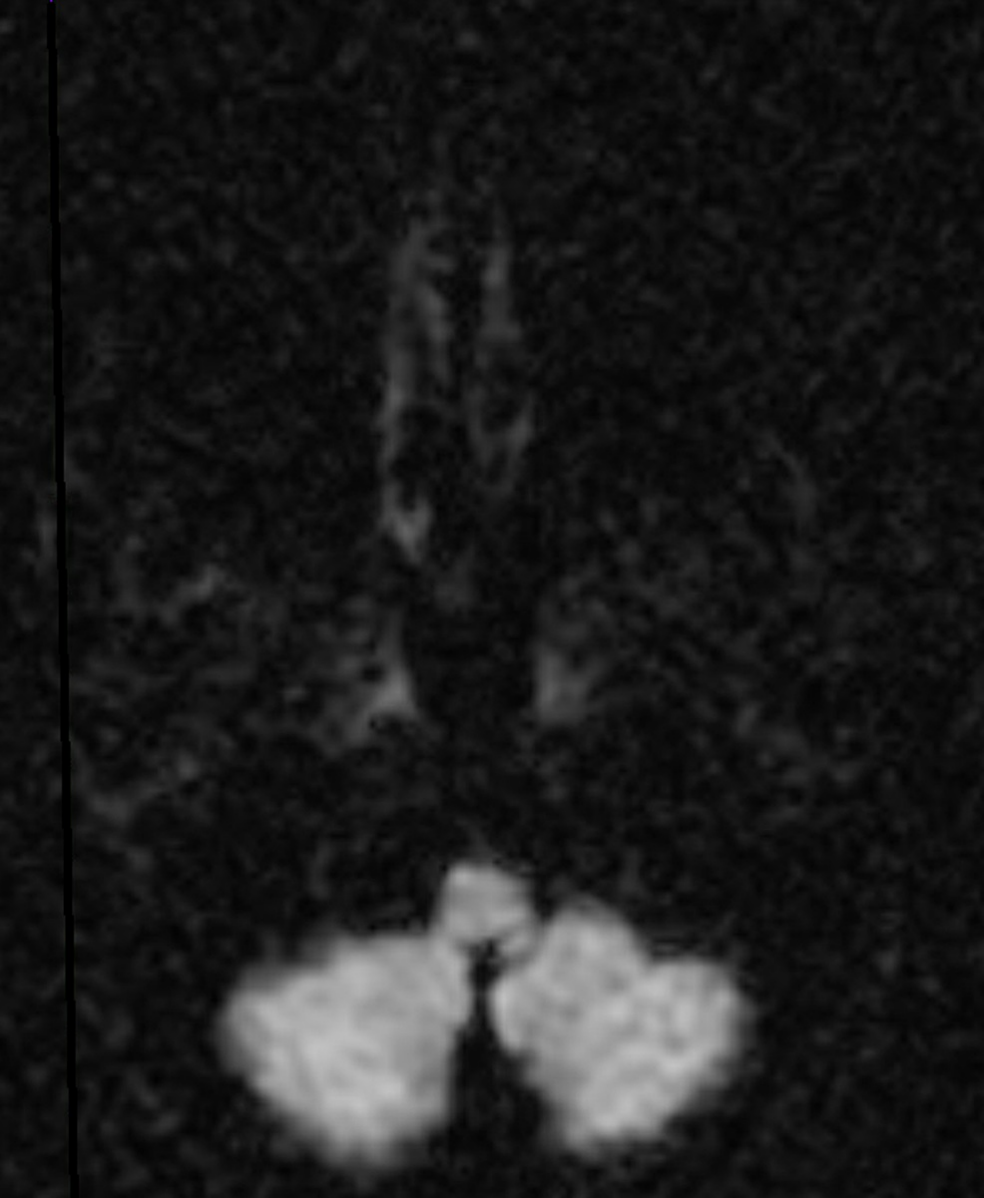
Initial MRI of the brain showing no significant hyperintensity on DWI DWI: diffusion-weighted imaging
